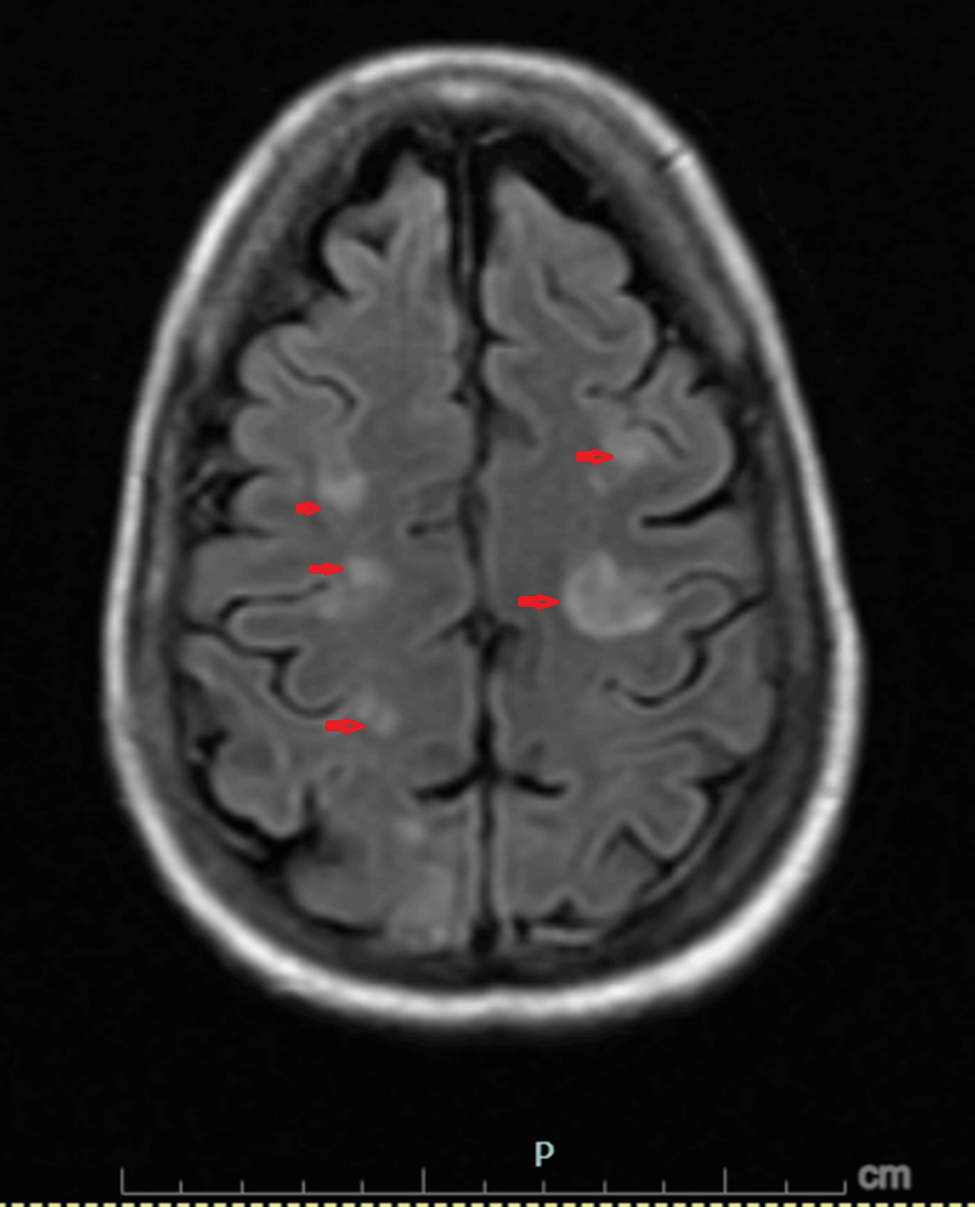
Cerebral involvement on brain MRI Magnetic resonance imaging (MRI) of the brain with fluid-attenuated inversion recovery (FLAIR) sequences demonstrating symmetric subcortical hyperintensities (red arrows) involving the bilateral cerebral hemispheres. There is no associated mass effect, diffusion restriction, or abnormal enhancement.
